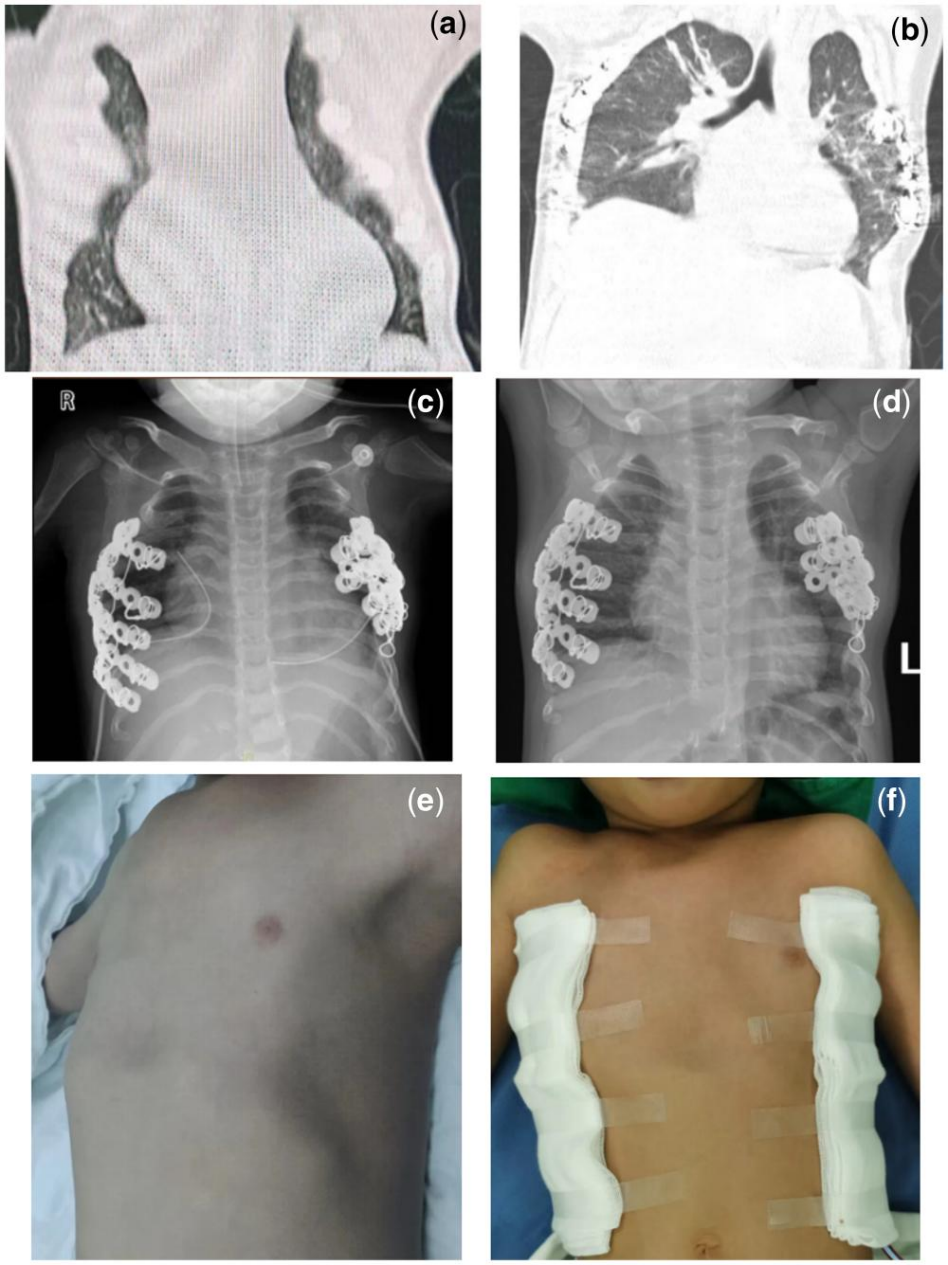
(a)Preoperative and (b)postoperative radiologic diagnosis using computed tomography; Radiologic diagnosis using digital radiology. (c) Four days postoperatively:1.Bilateral ribs show internal fixation with visible metal density. 2. light exudation in the lower left lung, suggestive of an inflammatory process. 3. The heart is mildly enlarged. (d) 93 days postoperatively: The lesions in both lungs were absorbed compared to before the operation; preoperative (e) and postoperative (f) thoracic photos.
